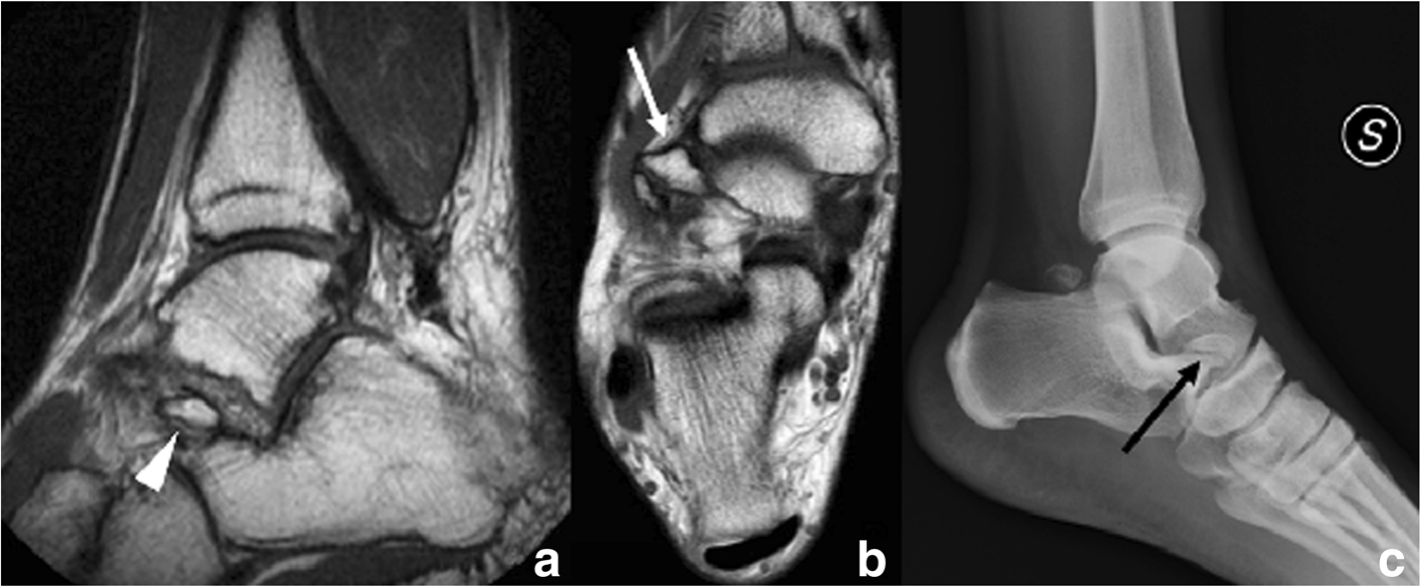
Os calcaneus secundarius. a Sagittal FSE T1 in a 56-year-old man referred for follow-up after Achilles reconstructive surgery. As an incidental finding, an os calcaneum secundarium was noted. This is located in between the talus and the calcaneus (white arrowhead). b Axial FSE T1 demonstrates the ossicle located in the space between the talus and calcaneus, articulating with the anterior process of the calcaneus (only partially seen) and the tarsal navicular (white arrow). c Different patient, incidental finding of an os calcaneus secundarius, here visible on lateral radiographs (black arrow). Note also the presence of an os trigonum
